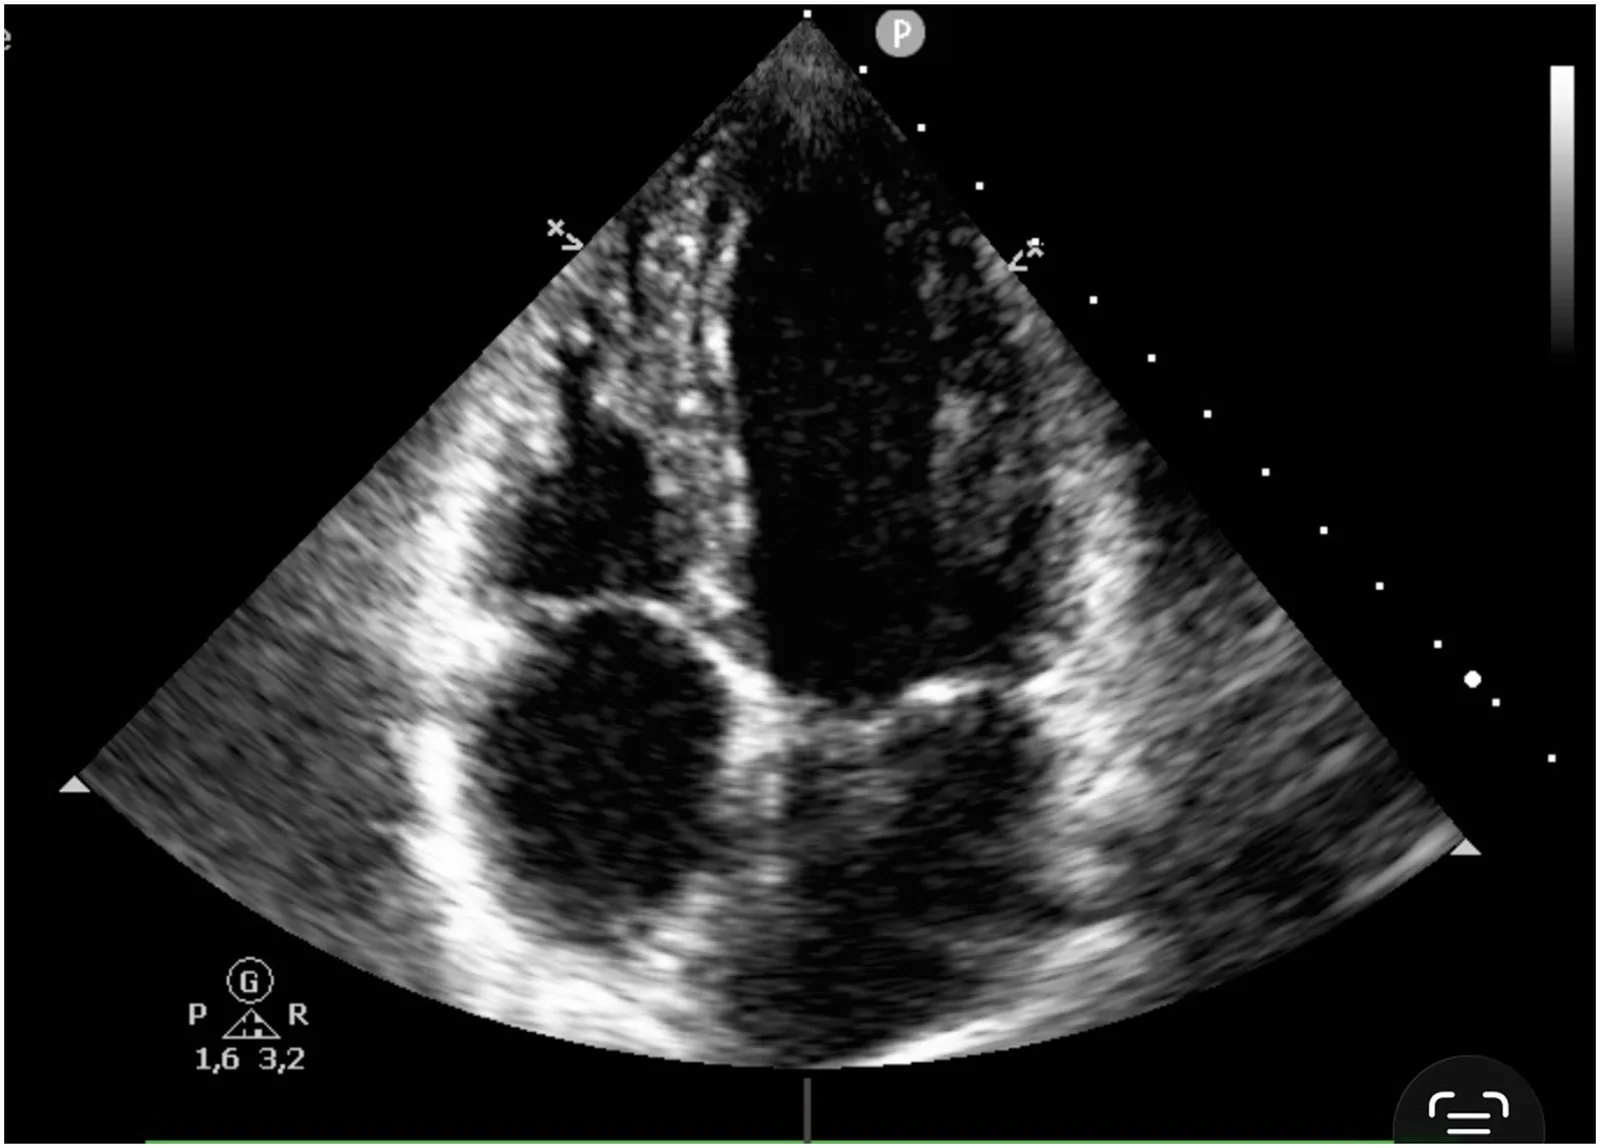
Follow-up transthoracic echocardiography after recovery. Follow-up transthoracic echocardiography at 8 months, obtained in the apical four-chamber view, demonstrating complete resolution of the previously documented left ventricular thrombi, normalization of left ventricular cavity appearance, and marked recovery of left ventricular systolic function, with left ventricular ejection fraction improving to 57%.
